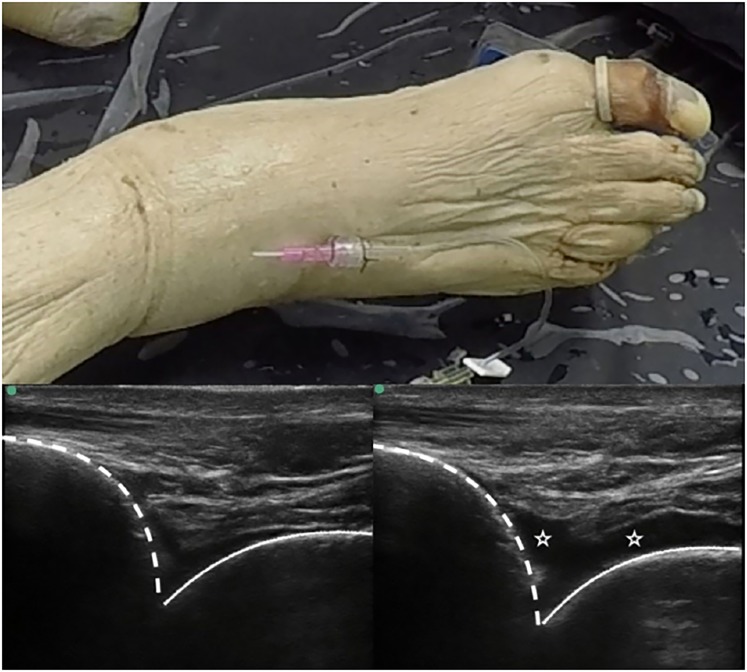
The catheter within the joint used to create effusion. Also shown are examples of an ankle pre- and post- effusion creation. The tibia (dotted line) and talus (solid line), as well as effusion (stars), are shown on ultrasound.
